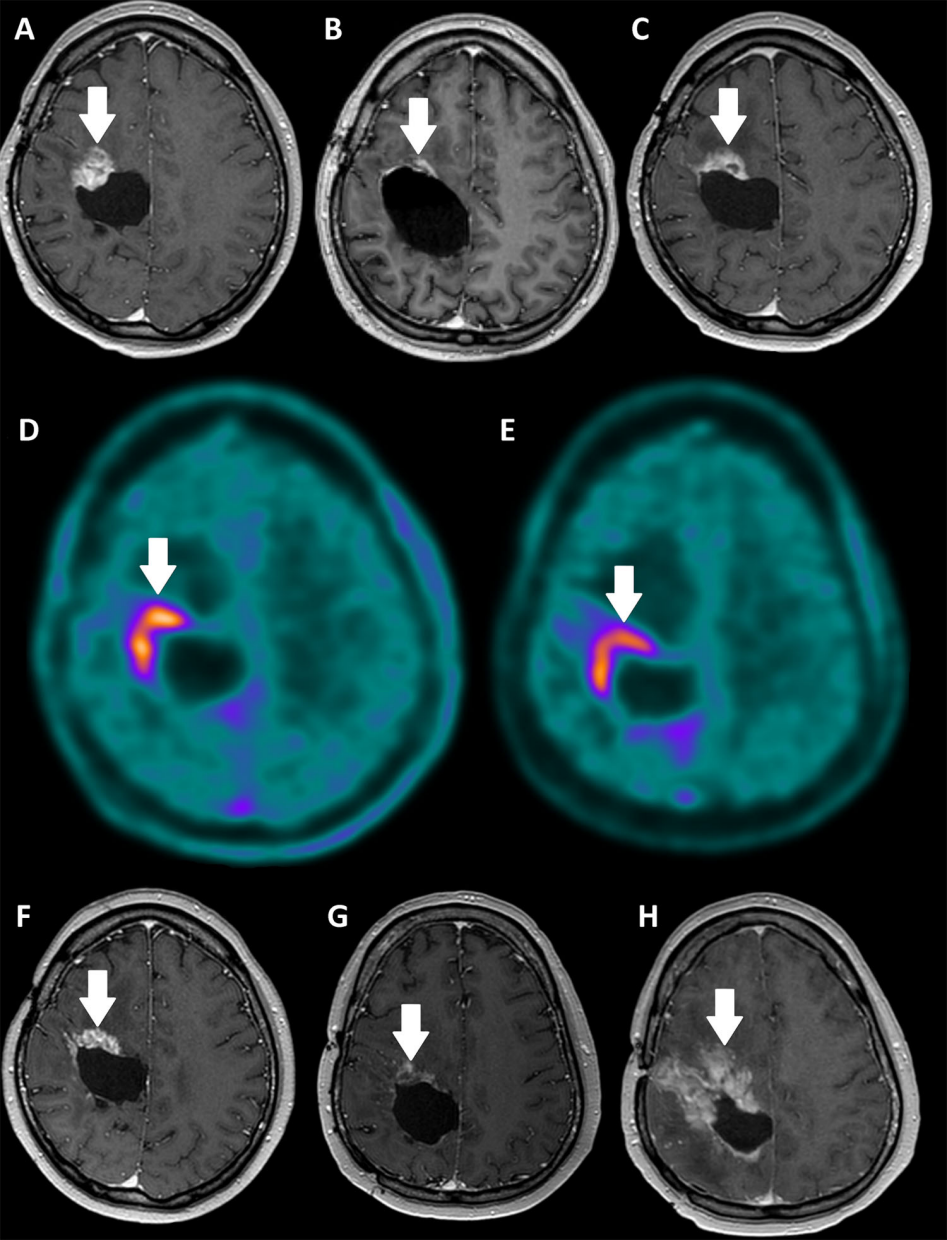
A Recurrent anaplastic oligodentritic astrocytoma, WHO grade 3, on a gadolinium-enhanced T1 (gdMR) sequence, shown as an enhancing mass on the anterior side of the primary resection cavity. B First postoperative gdMR 4 days after surgical re-resection, showing postoperative rim contrast enhancement in the operation cavity. C Follow-up gdMR 3 months after surgical re-resection, demonstrating a recurrent mass in the same location. D Flour-18-ethyltyrosine ([^18^F]FET) PET/CT demonstrating pathological uptake (tumor-to-background ratio of 3.2), confirming tumor recurrence and demonstrating patients eligibility for treatment with iodine-131-phenylalanine ([^131^I]IPA)–Theranostic approach. E [^18^F]FET-PET/CT 3 months after two cycles of [^131^I]IPA (once intravenous and once intra-arterial), demonstrating stable disease according to PET RANO criteria, but with minor decrease in [^18^F]FET uptake. F gdMR 3 months after two cycles of [^131^I]IPA, demonstrating decreased contrast enhancement, stable disease according to RANO criteria. Because of clinical deterioration, at multidisciplinary tumor board (MDT), remaining contrast enhancement on gdMR was deemed to be partially radiation necrosis, for which bevacizumab treatment was initiated. G gdMR after two cycles of bevacizumab, demonstrating near complete response with small areas of contrast enhancement remaining. Thus, MDT deemed the interim diagnosis of radiation necrosis valid. H) Multifocal progressive disease nearly 12 months after the two [^131^I]IPA treatments, with clear progression at the same location and new lesions in-cerebro (new lesions not shown). One month later, 13 months after the second cycle of [^131^I]IPA, patient died
